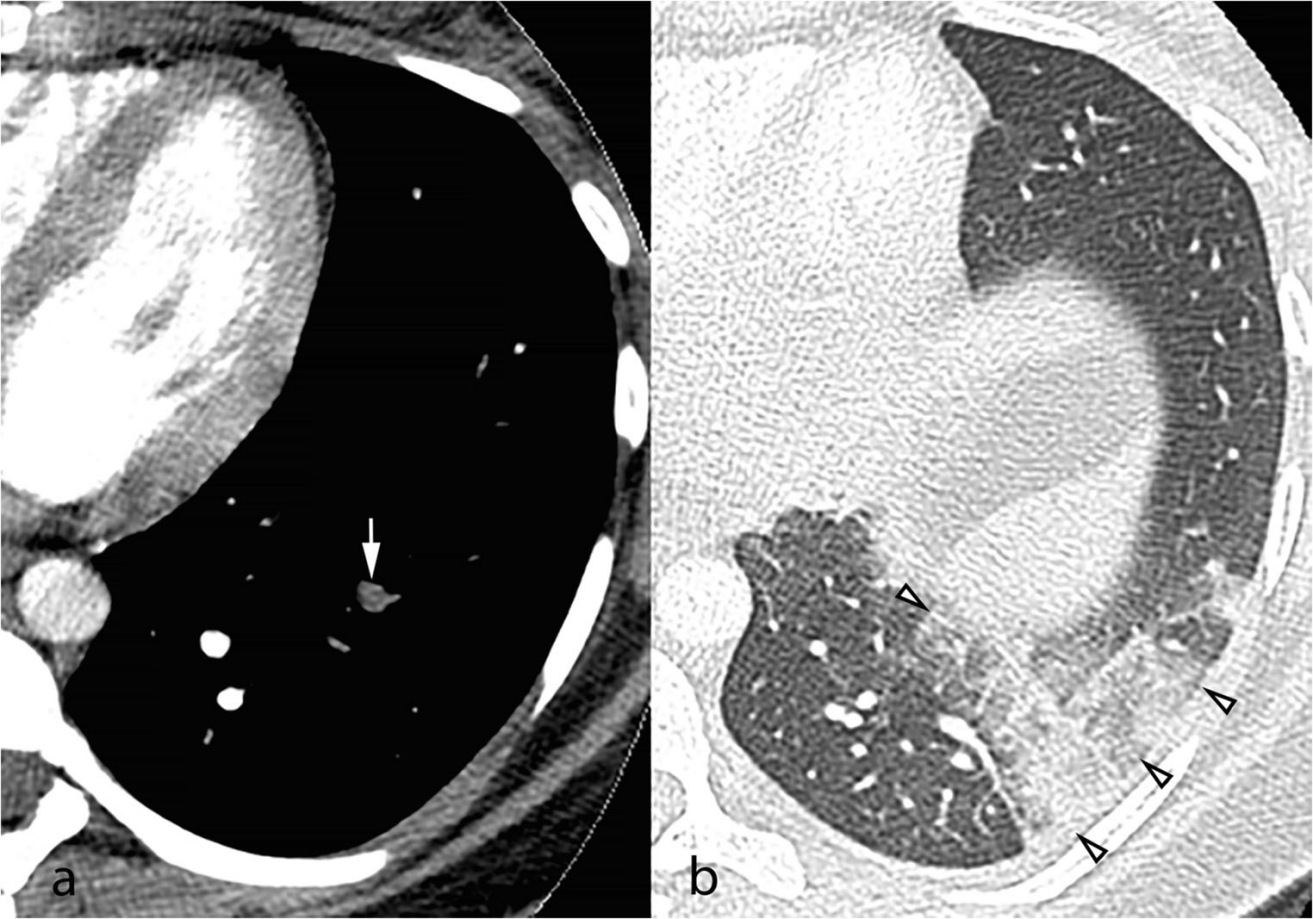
Axial computed tomography pulmonary angiography images in the soft tissue (a) and lung windows (b), from a 25-year-old pregnant patient presenting with acute chest pain. A segmental pulmonary embolus was detected in the left lower lobe (a, white arrow), with associated triangular-shaped areas of subpleural alveolar density (b, arrowheads), consistent with pulmonary infarction
